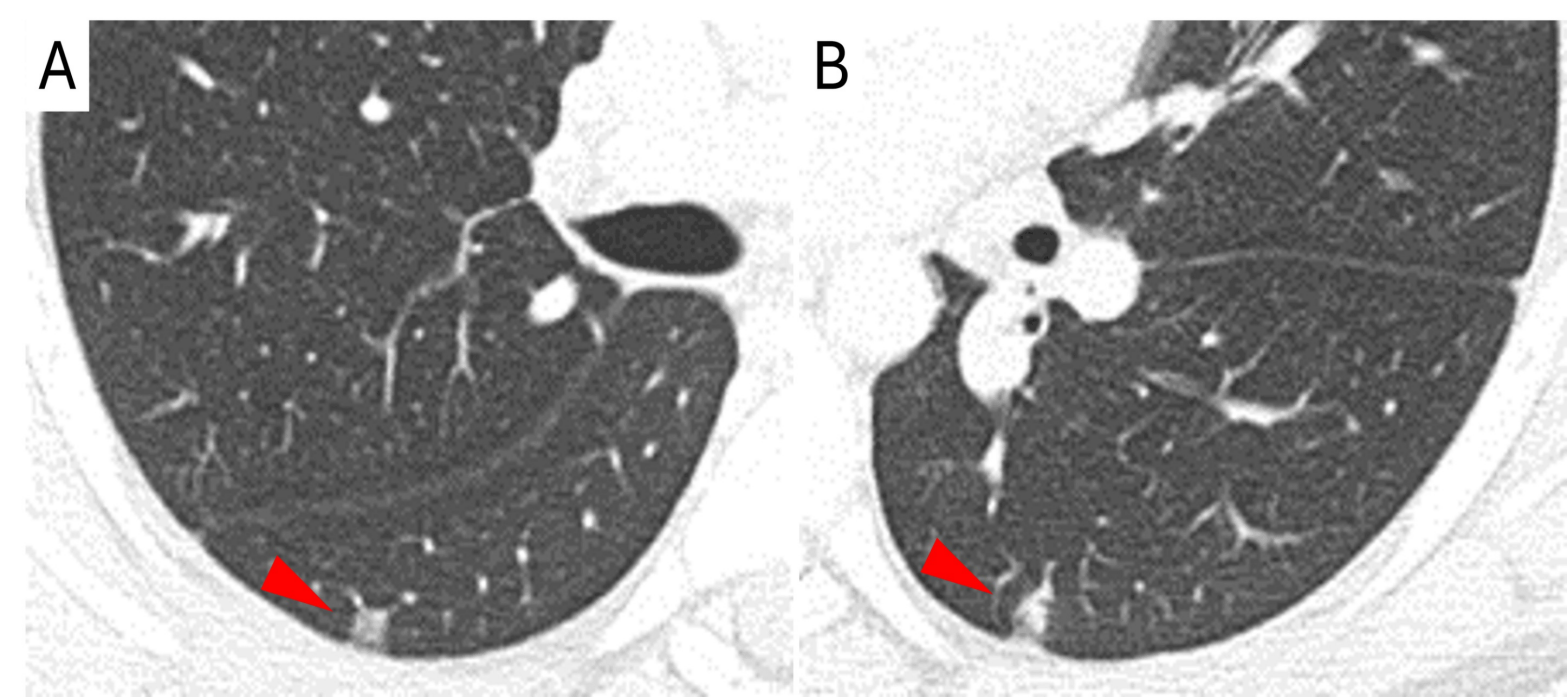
CT of the chest without contrast. CT of the chest without contrast on the day of admission revealed two wedge-shaped small nodules (arrowheads) in both right (A) and left (B) lung bases. CT: computed tomography.
